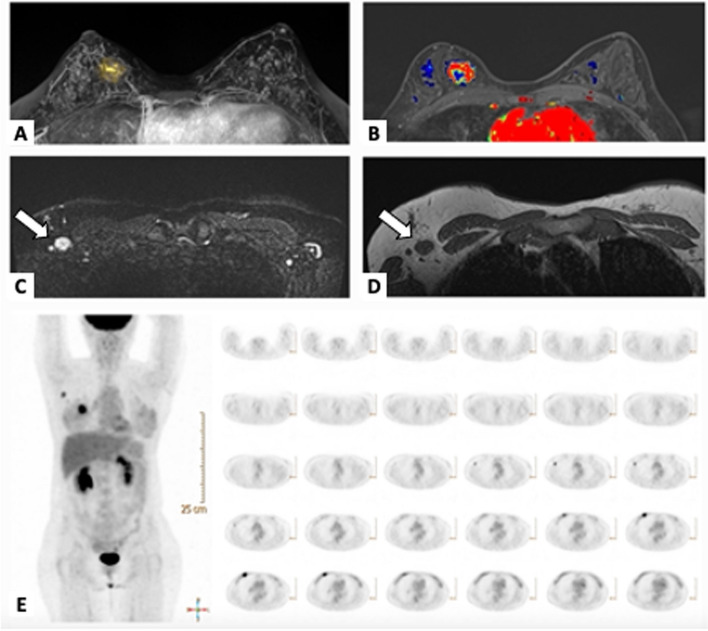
Patient with a concordance between imaging results and pathological results. A 51-year-old female patient with invasive ductal carcinoma in the upper inner quadrant of the right breast. A–B Breast MRI depicted the round, heterogenous enhancing tumor, between 12 and 2 o’clock, middle-posterior, and 1/3 in depth, approximately 3.2 cm in assessment. T2WI and T1WI images showed a level 1 node (C–D, arrow). PET/CT showed intense FDG hypermetabolism in the right breast (*SUV*: 7.5 on early images) (E) suggesting right breast malignancy and a nodular FDG hypermetabolism in the right axilla (*SUV*: 3.8), suggesting right axillary lymphadenopathy. The final surgical pathology showed invasive ductal carcinoma with a metastatic lymph node (1/23)
